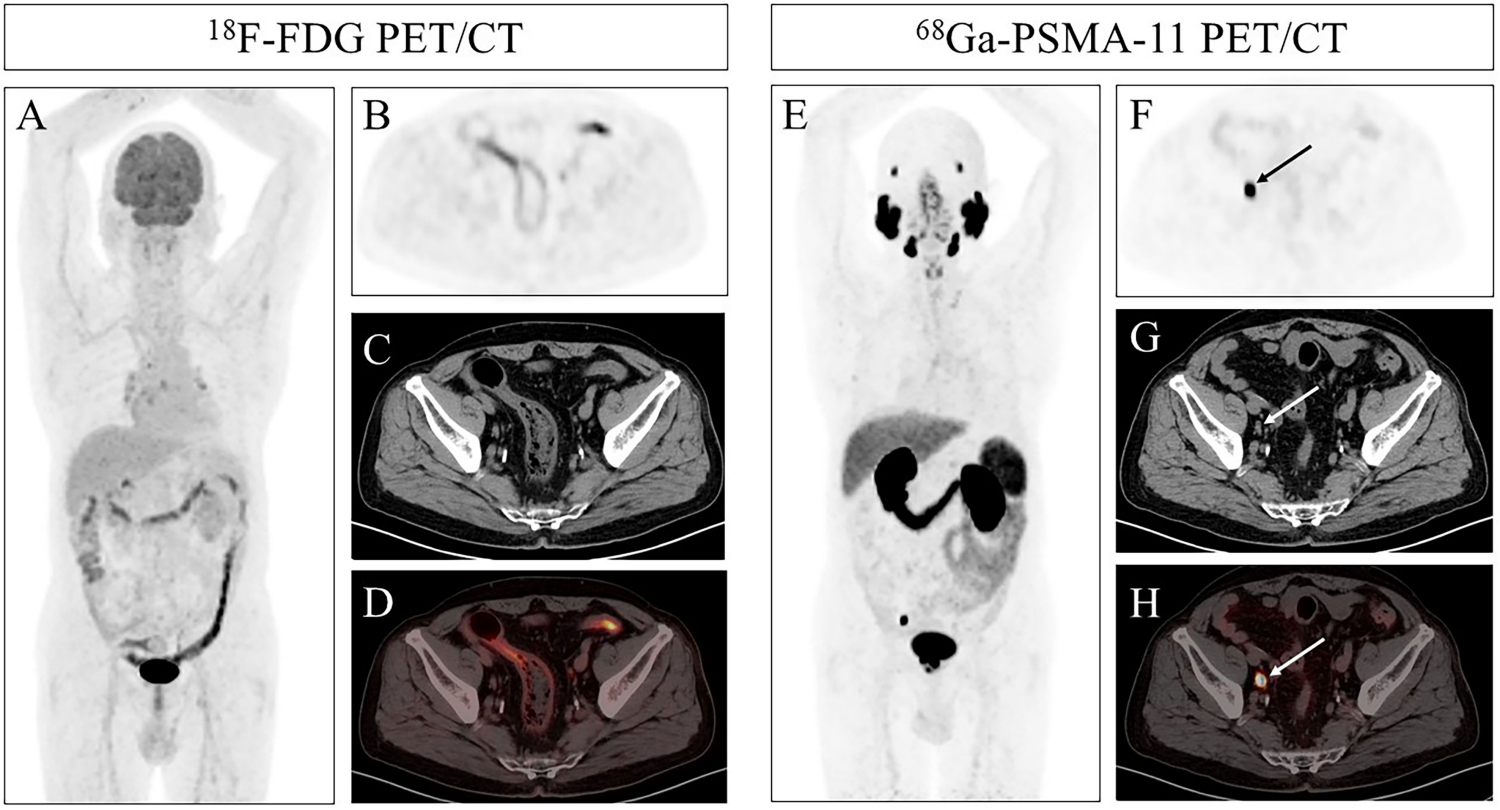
(Patient 21) An 80-year-old man presented with a 2-year history of progressive voiding difficulty and a serum PSA of 30.70 ng/ml. ^18^F-FDG PET/CT MIP and axial images (A, B) showed no abnormal FDG uptake in the right external iliac region. Corresponding CT and fused PET/CT slices (C, D) confirmed the absence of hypermetabolic lymphadenopathy. ^68^Ga-PSMA-11 PET/CT MIP and axial views (E, F) revealed intense PSMA avidity in a right external iliac lymph node (solid arrow; SUVmax 15.51). (G, H) CT and fused PET/CT images precisely localized the right external iliac lymph node metastasis, which was validated by follow-up imaging. Multiple lymph nodes, the left lobe of the liver, and the splenic parenchyma showed no corresponding increase in FDG uptake (black arrow).
